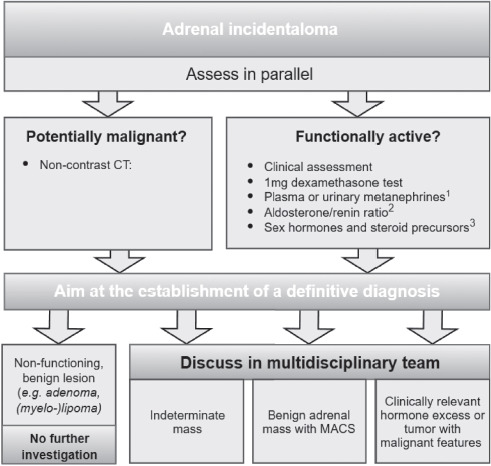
Flow-chart on the management of patients with adrenal incidentalomas, adapted from European Society of Endocrinology 2023 guidelines. ^1^Only necessary in adrenal tumors with Hounsfield unit (HU) > 10 in unenhanced CT. ^2^Only in patients with concomitant hypertension and/or hypokalemia. ^3^Only in patients with clinical, biochemical, or imaging features suggestive of adrenocortical carcinoma [6].
